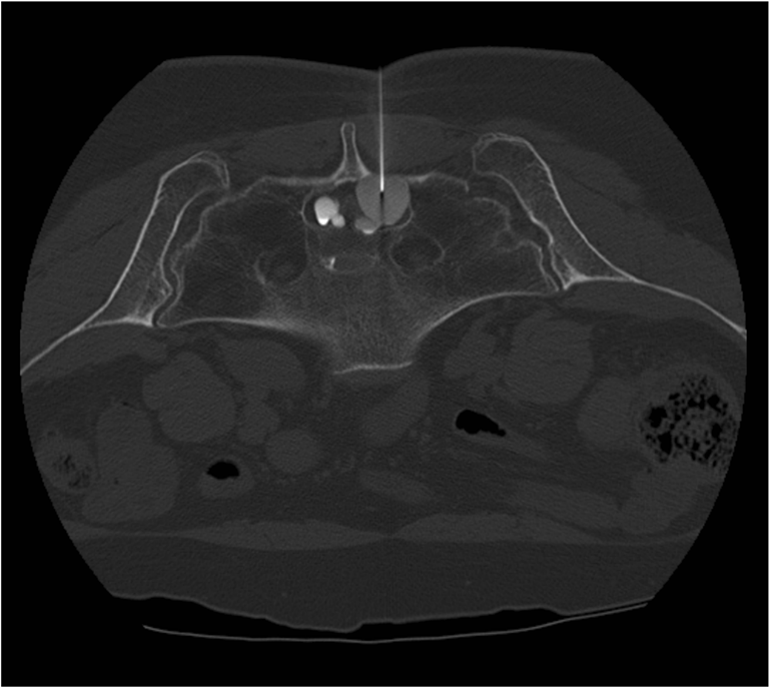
Axial computed tomography scan showing percutaneous aspiration of a sacral perineural cyst
